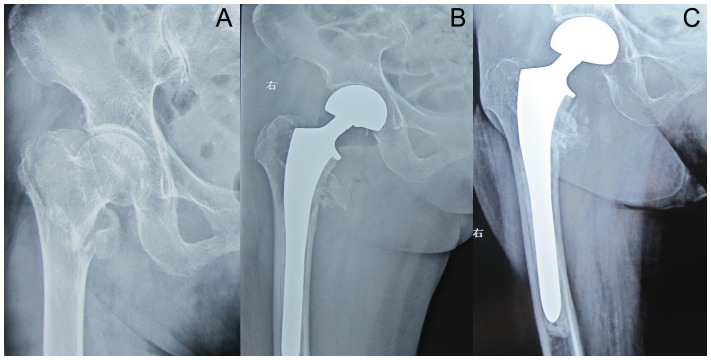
AP hip radiographs of a 78-year-old woman with severe osteoporosis and unstable intertrochanteric fracture treated with cement bipolar hemiarthroplasty using a long stem. A: Preoperative; B: At 6 months postoperatively, the fractured fragment has healed; C: radiographs obtained three years postoperatively, Femoral component was stable with no protrusion of cup.
